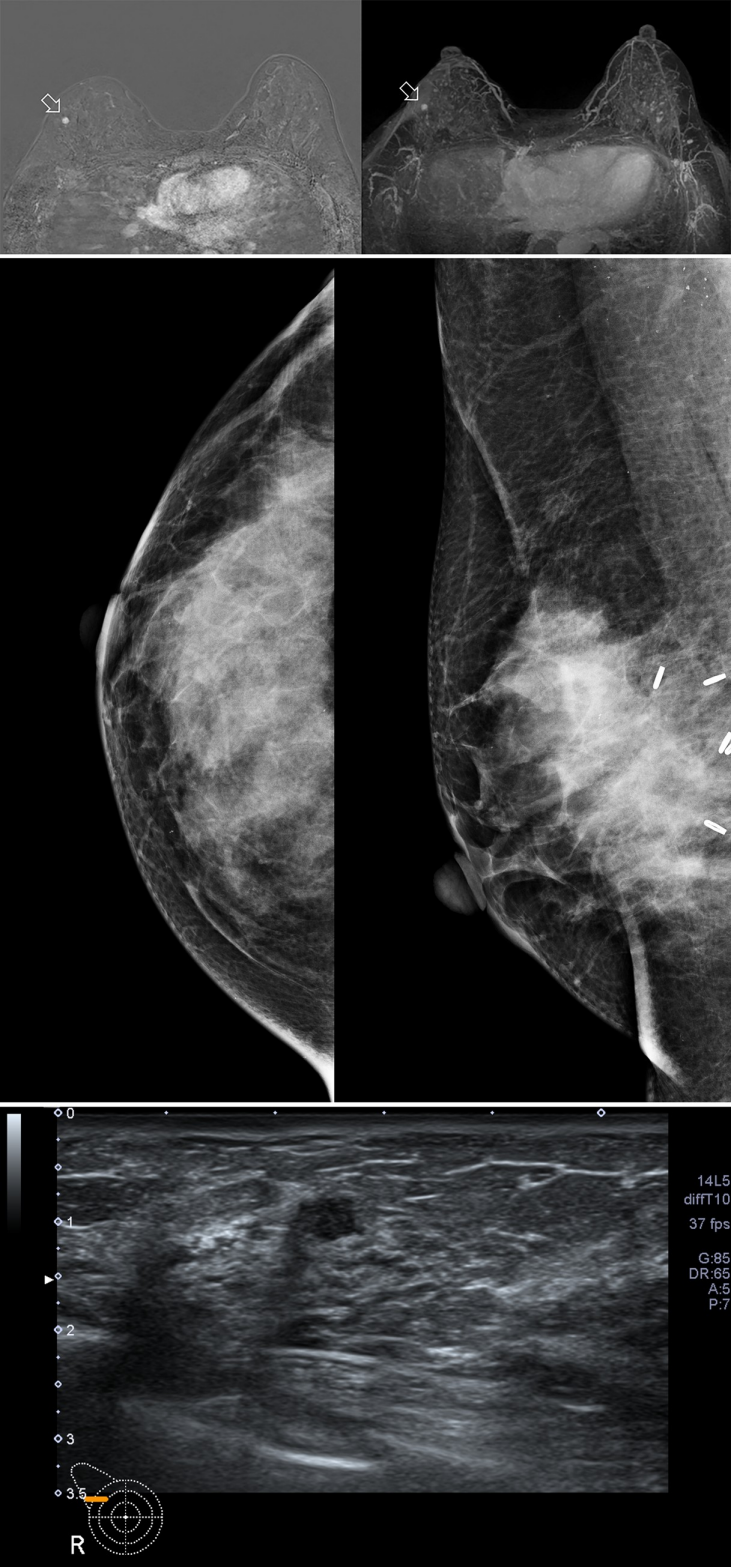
A 43-year-old female patient with a late recurrent breast cancer detected 20 months after BCT. (a) MRI of a 43-year-old patient who underwent BCT for right breast cancer (invasive ductal carcinoma, stage II). The patient underwent BCT alone and refused radiation therapy and chemotherapy after surgery. On the second annual postoperative MR surveillance, a small (0.6cm) sized enhancing nodule is detected at the upper outer quadrant of right breast (10 o’clock direction, open arrow). (b), (c) Mammography and ultrasound of the same patient for routine postoperative surveillance. There is no suspicious finding on mammography. On the second look ultrasound, there is a small hypoechoic nodule at the possible concordant location with the MR detected lesion. This lesion was underestimated before MRI examination due to multiple hypoechoic nodules at upper outer quadrant of right breast. It was confirmed as recurrent invasive ductal carcinoma by core needle biopsy.
